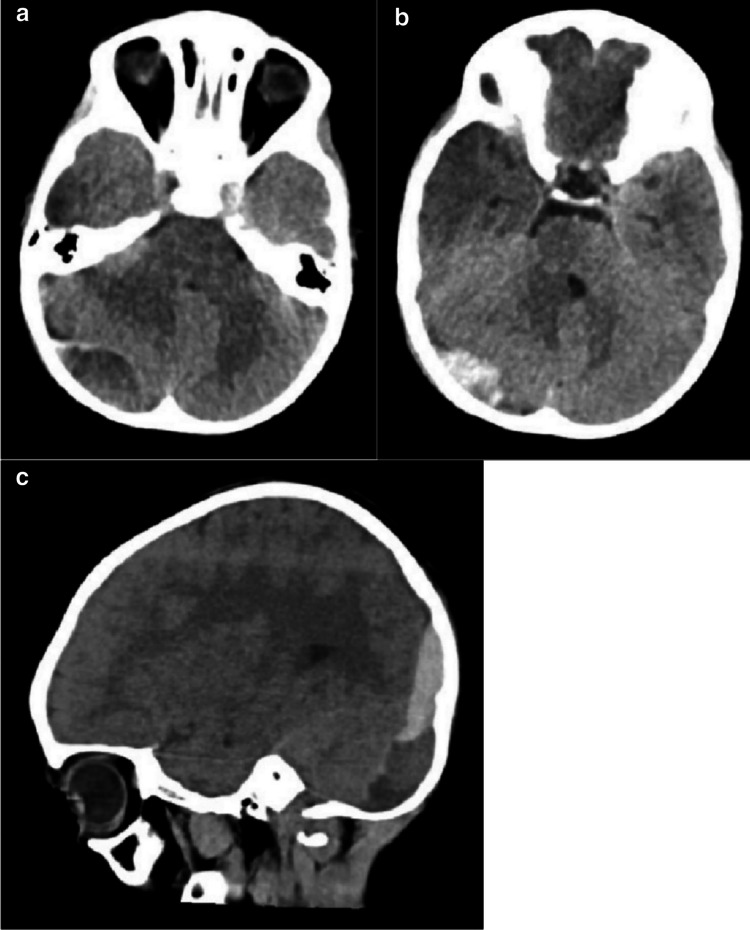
a and b Axial and c sagittal non-contrast enhanced CT images of acute extradural haematoma in the right posterior fossa on presentation.
